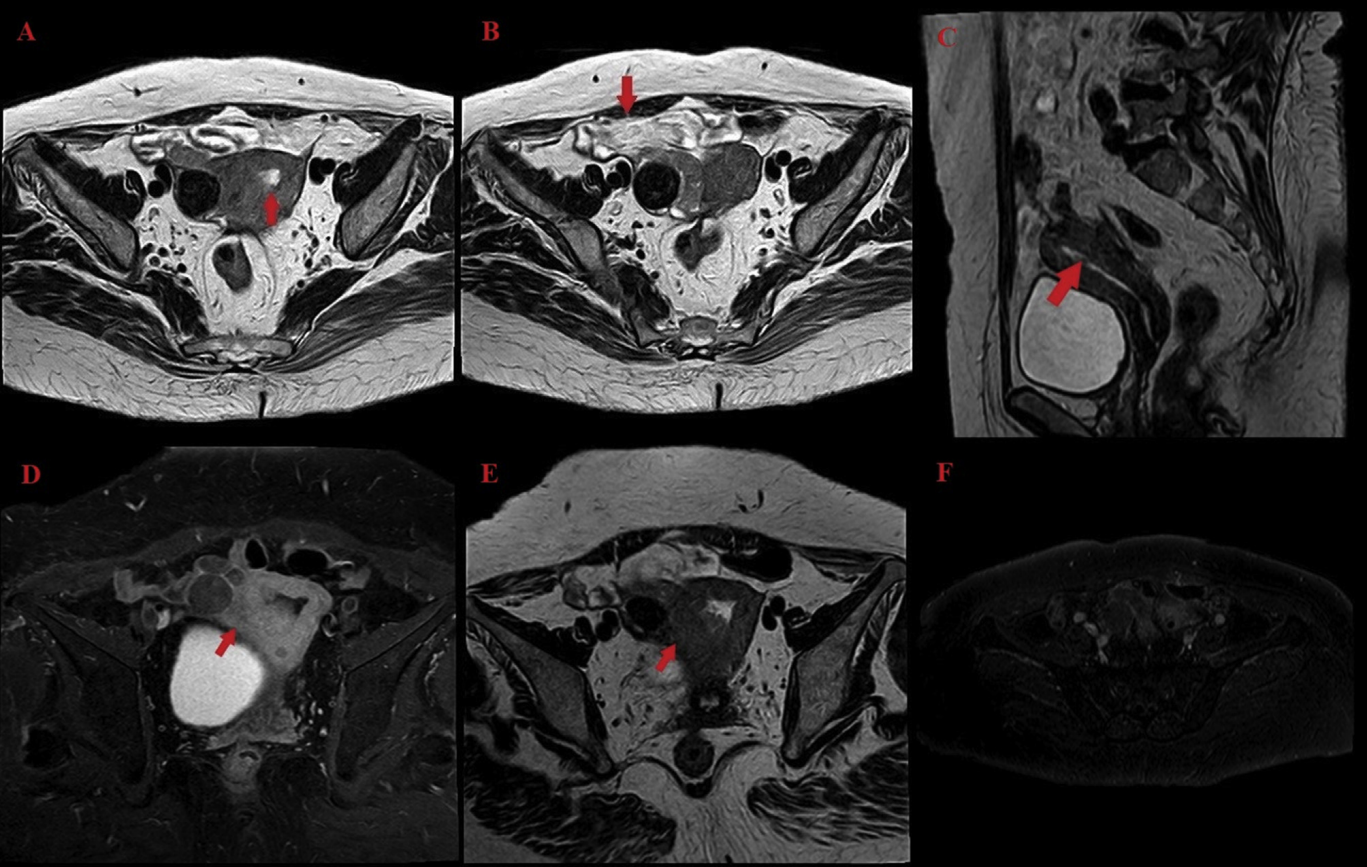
MRI images. (A and B) Axial view showing tumor mass inside the endometrium and at the right fallopian tube. (C) Sagittal view of endometrial tumor. (D and E) T2 and T1 image that showed mild enhancement from contrast administration. (F) Axial view of contrast enhancement.
